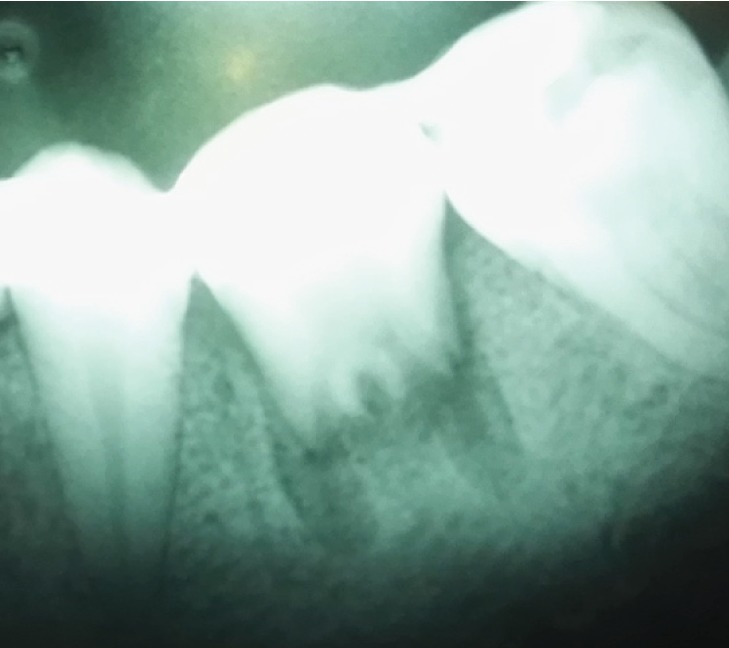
Immediate postoperative radiograph showing third molar fitting well in tooth number 19 socket.
